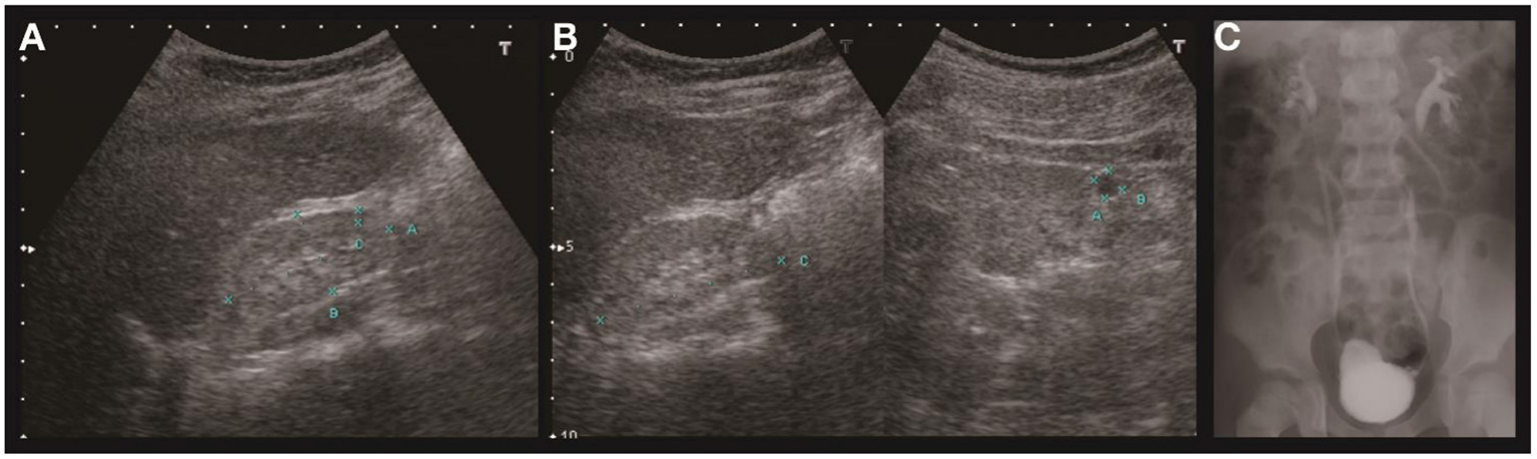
Renal ultrasound and voiding cystoureterography of case A. Sonography of the right (A) and left (B) kidney revealed hypoplasia and hyperechogenicity of bilateral kidneys. The right kidney measured 4.6 cm in length and left kidney 4.9 cm. A cyst was found in the left kidney. Voiding cystoureterography (C) revealed bilateral vesicoureteral reflux (right side grade II, left side grade III).
